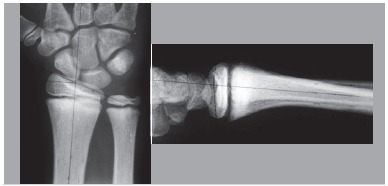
Front and profile x-ray of the left wrist of a young athlete without complain of wrist pain Observe the marks at ulnar (20º) and radial (9º) inclinations, respectively.
